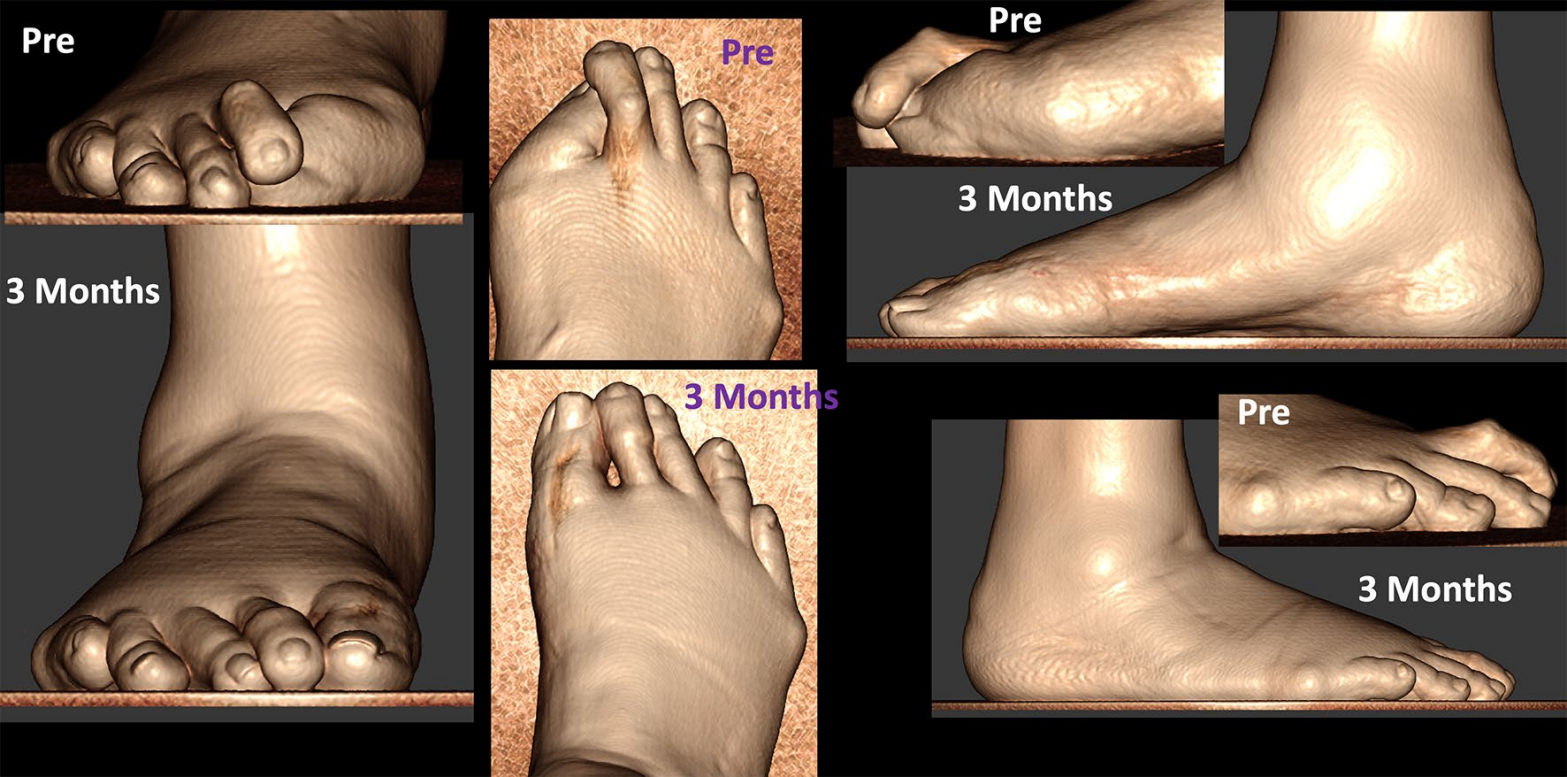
Tree-dimensional surface soft tissue anatomy weight bearing CT reconstruction views of patient’s foot, demonstrating preoperative severe hallux valgus deformity and cross-over lesser 2nd toe deformity, and considerable correction 3-months postoperatively
